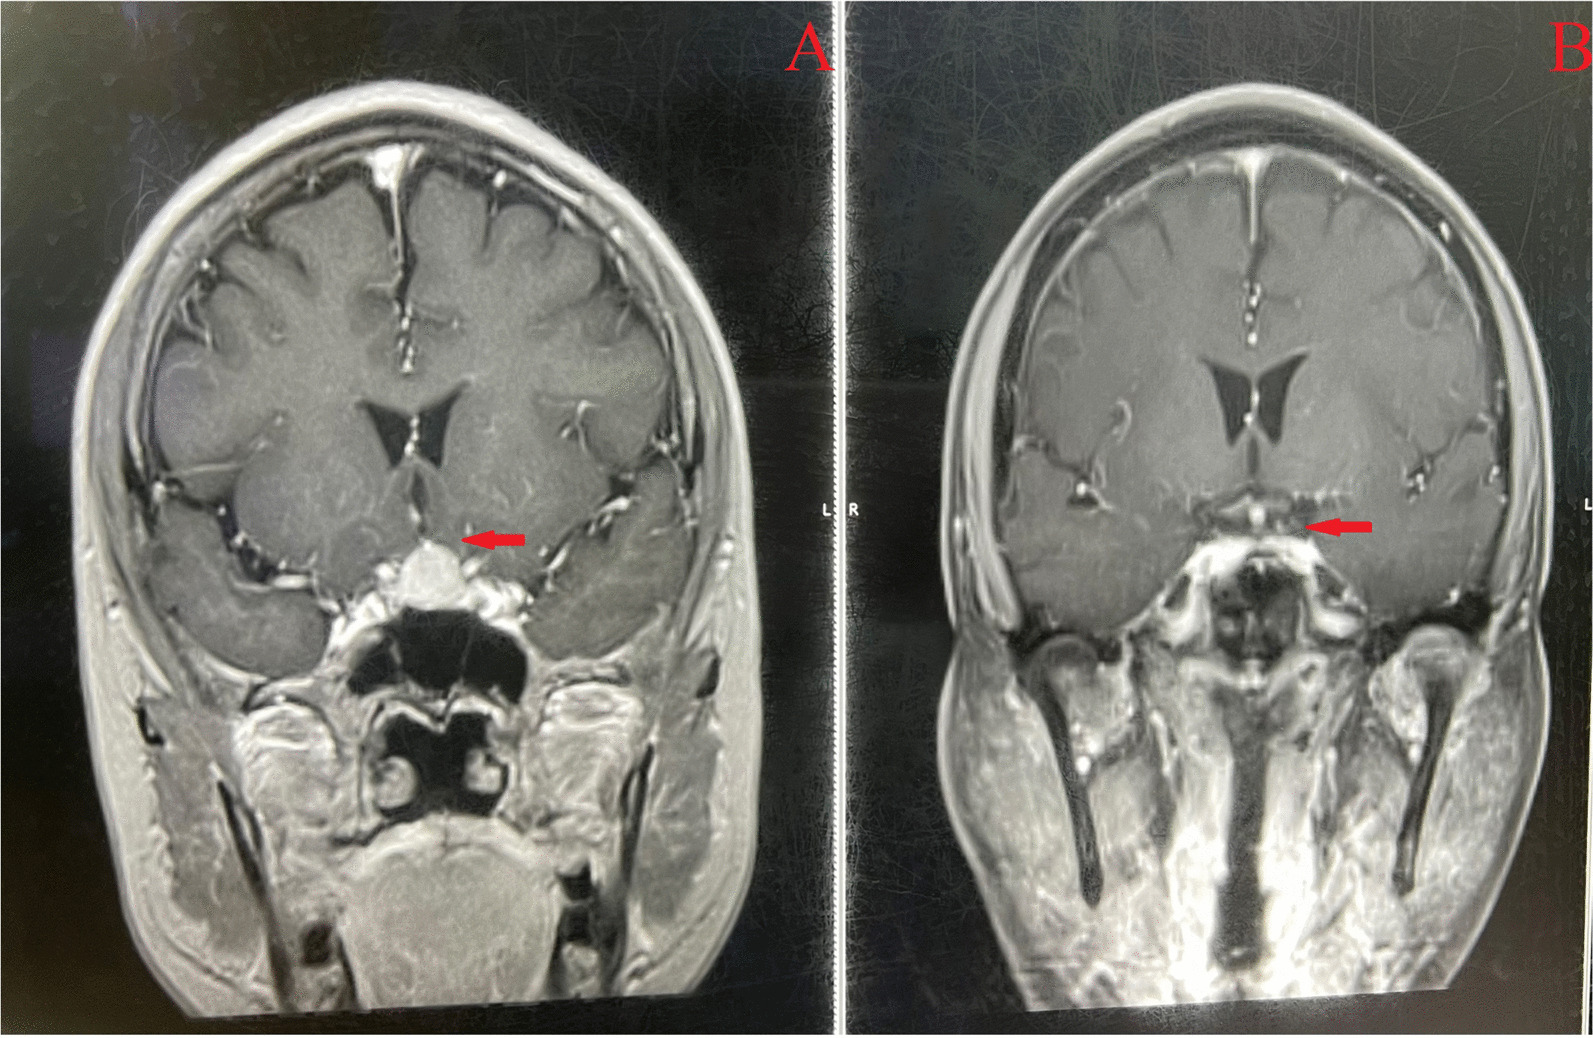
Images from the craniocerebral magnetic resonance imaging scan of case 2. A Shows the craniocerebral magnetic resonance imaging scan of case 2. B Shows the craniocerebral enhanced magnetic resonance imaging picture of case 2 after treatment. The red arrow indicates the lesion site in the stellar region
